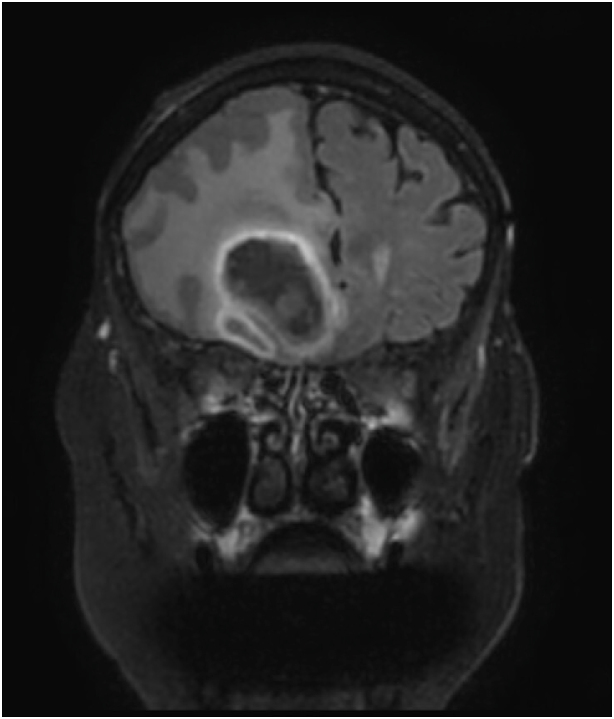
Brain MRI frontal section showing the multi-loculated right frontal lesion with important vasogenic edema.
